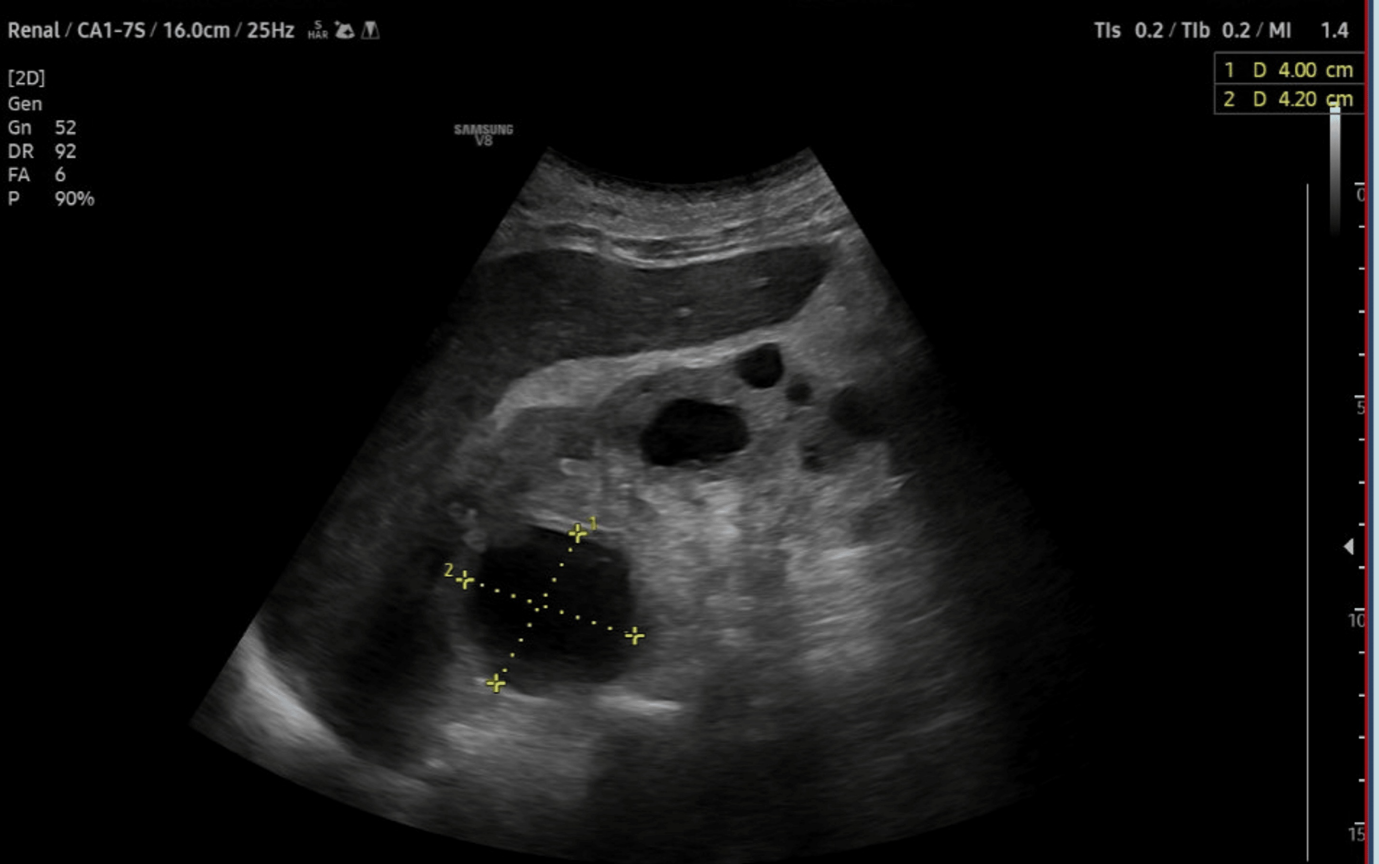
Renal ultrasound images showing renal cortical cysts.
